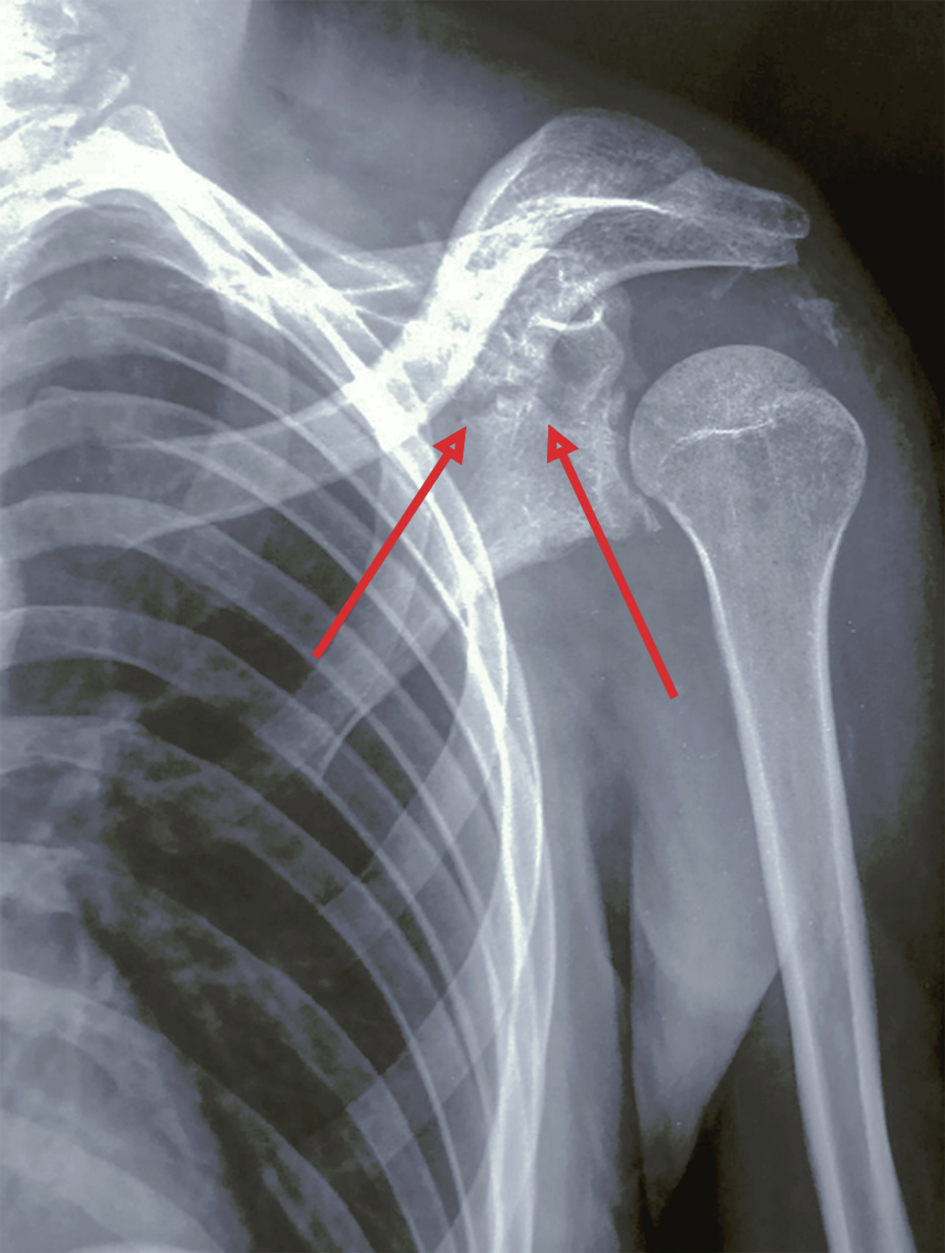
Anteroposterior radiograph of the left shoulder showing an Ideberg type III glenoid fracture
